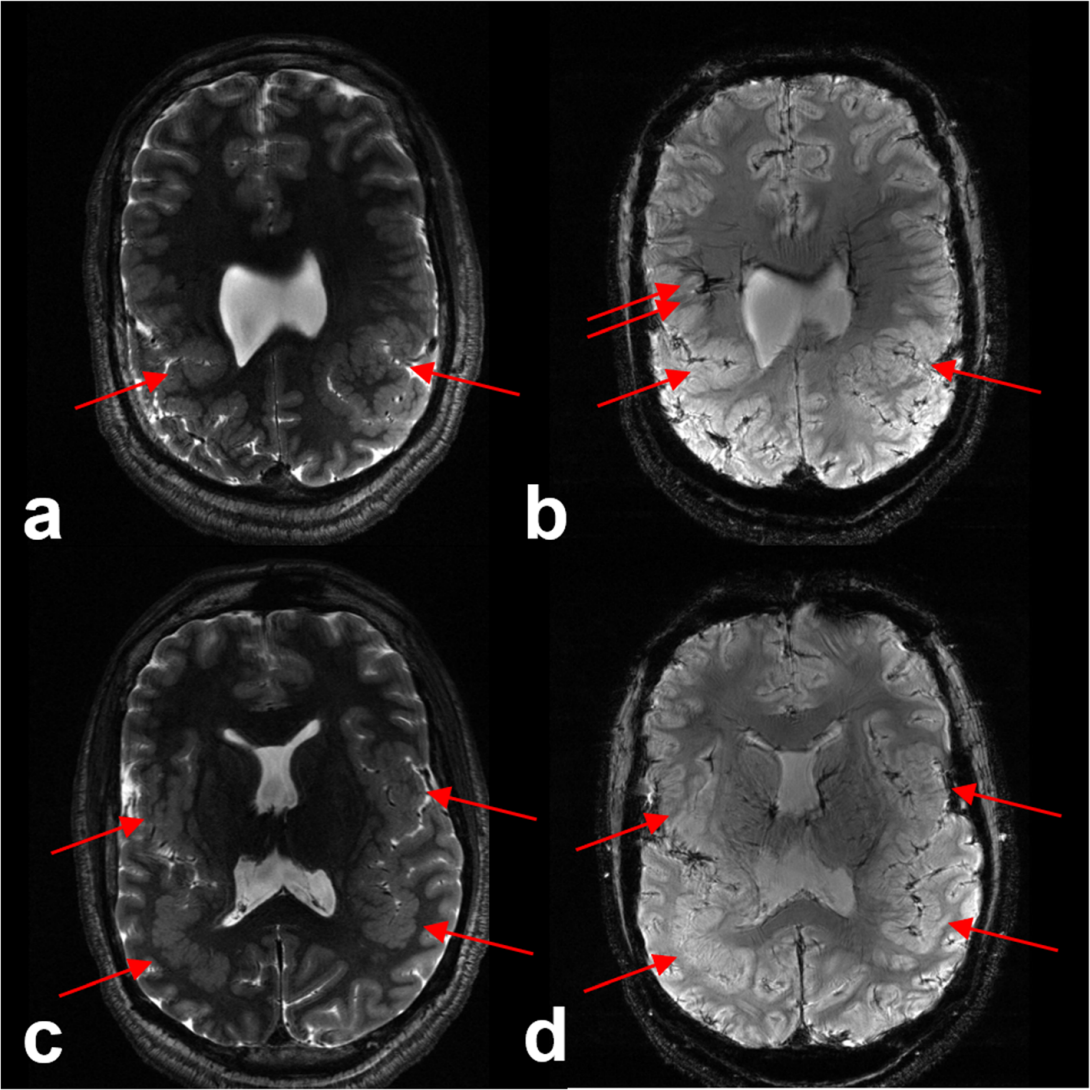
Sensitivity of 7T magnetic resonance imaging for polymicrogyria. Axial T2-weighted and susceptibility-weighted images at the level of the centrum semiovale (a, b) and lateral ventricles (c, d) demonstrate simplification of the gyral folding pattern involving the perisylvian cortex bilaterally, including the posterior temporal lobes and parietal lobes (red arrows), with thickening of the cortex and nodularity, consistent with polymicrogyria. There is also an abnormally large draining vessel on the right (b, red arrow), which may represent a developmental venous anomaly
